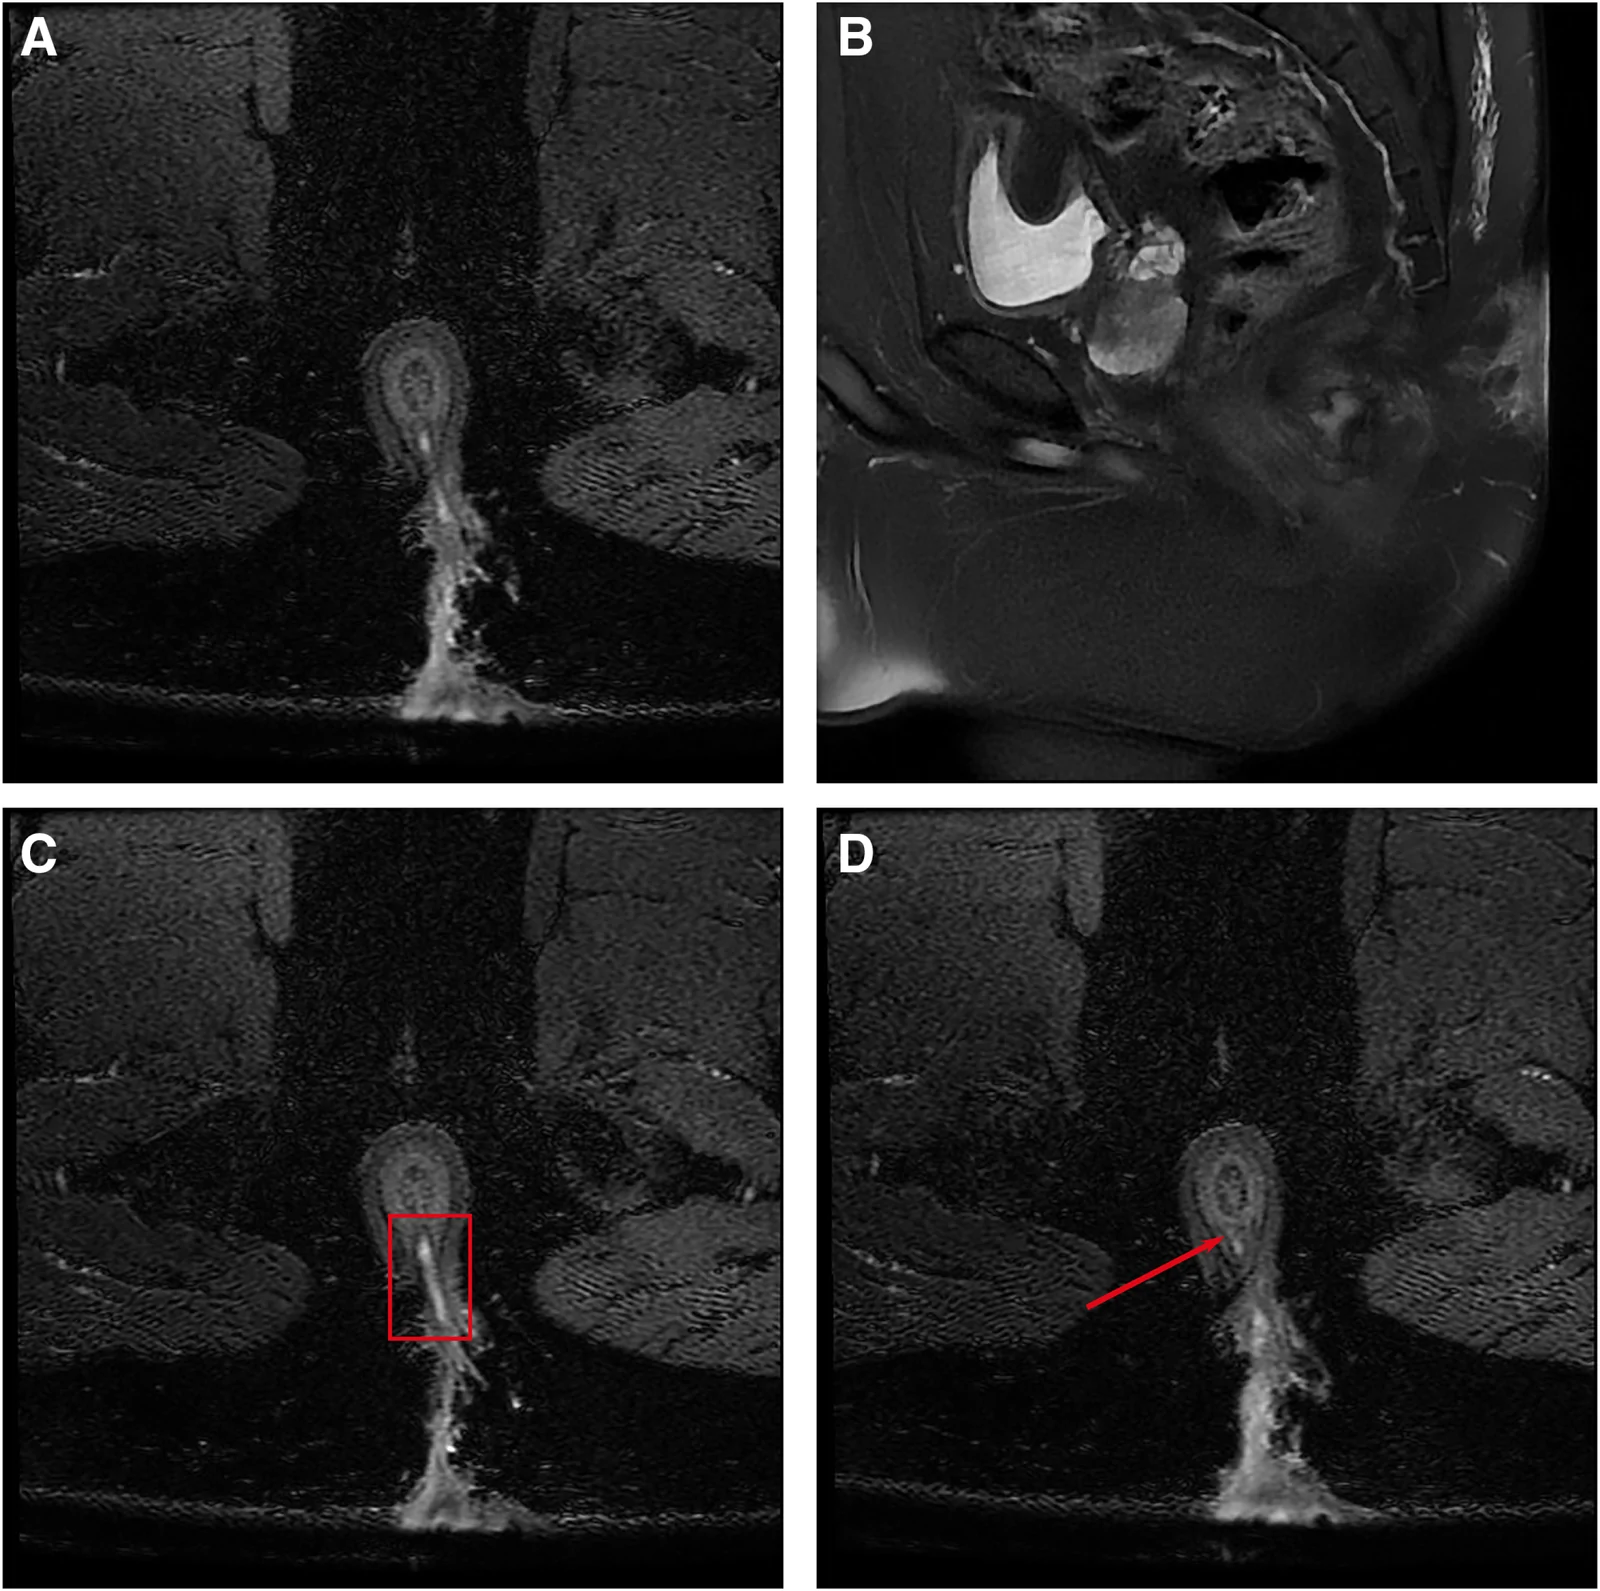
Follow-up pelvic MRI after wound debridement. Panels (A), (C), and (D) are oblique axial diffusion-weighted images, and panel (B) is an oblique sagittal T2-weighted STIR image. (A, B) Images showing near-complete resolution of the artifact. (C) Image showing the course of the fistulous tract (red rectangle). (D) Image showing the location of the internal opening of the anal fistula (red arrow). MRI = magnetic resonance imaging, STIR = short tau inversion recovery.
